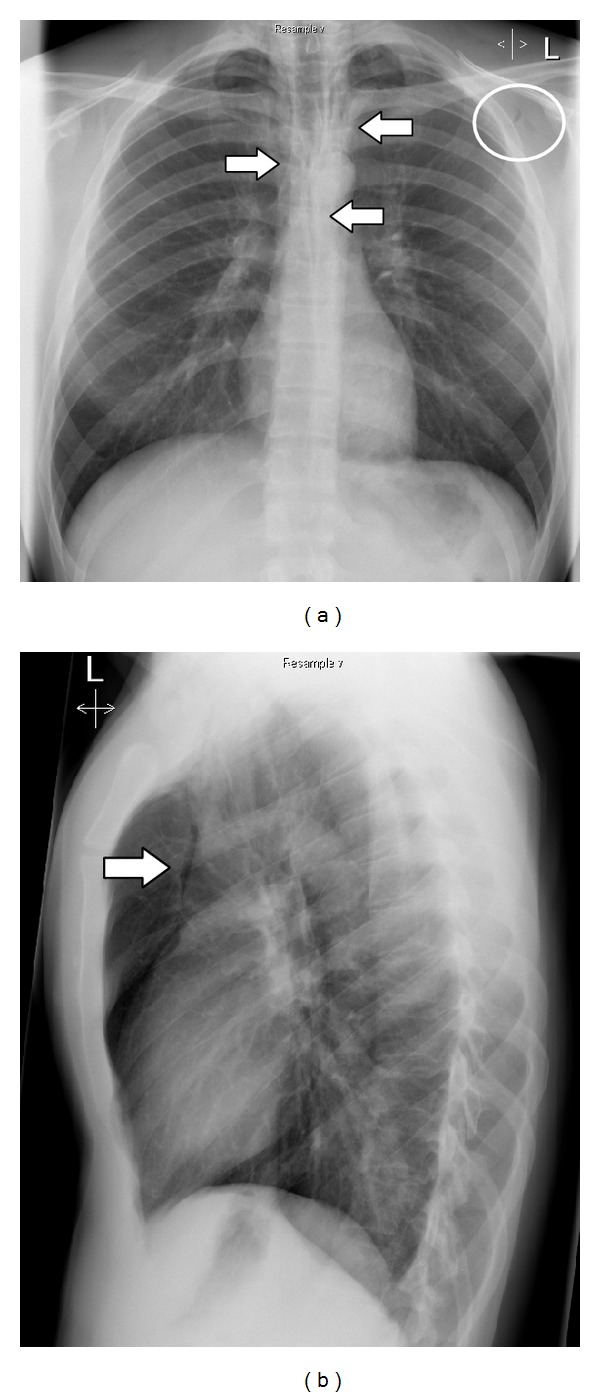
Chest X-ray showing air outlining mediastinal structures (arrows) and subcutaneous emphysema in the area of the left axilla (circle).
